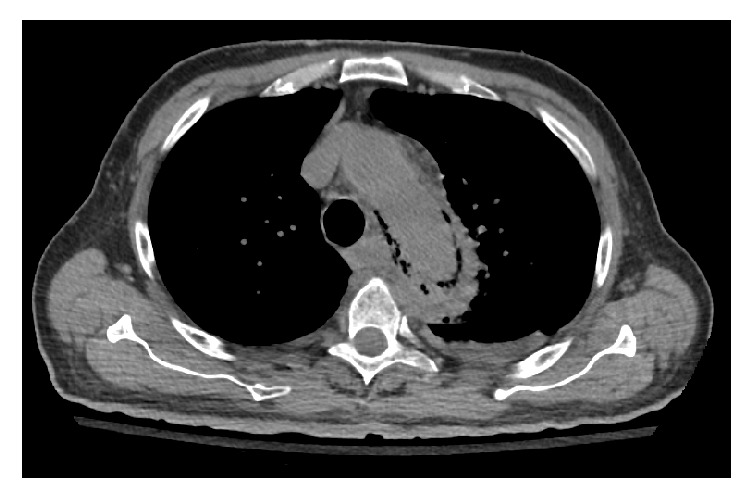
CT chest (sagittal view) showing emphysematous aortitis.
